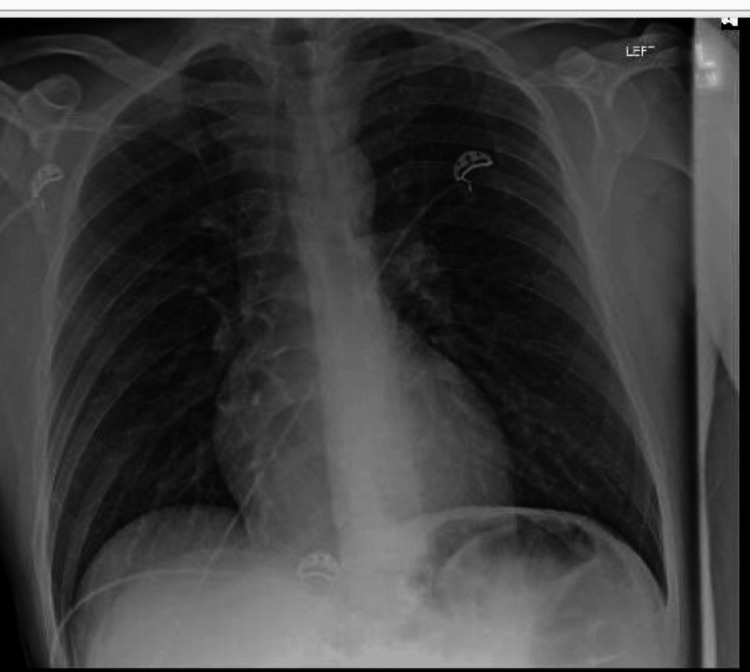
Posteroanterior (PA) view of the patient's chest X-ray
